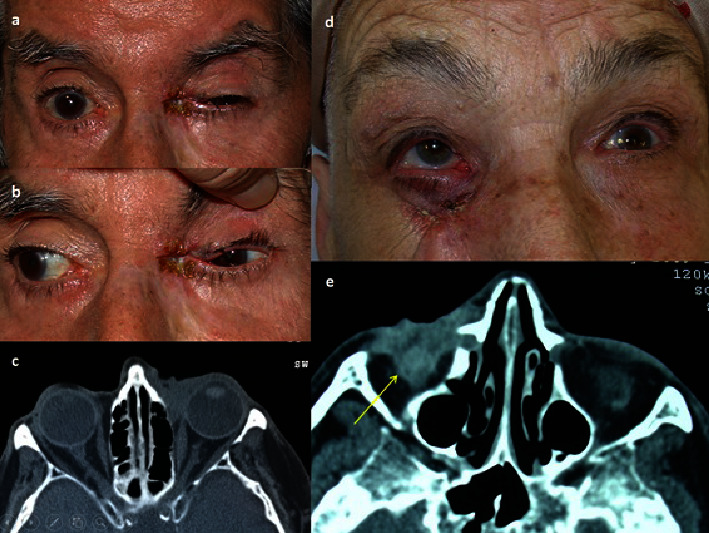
Recurrent basal cell carcinoma (BCC) (patient 11 in Table 1). (a) Ulcerative lesion in the medial canthus, adherent to deep planes. (b) Contraction of the eyelids that makes them difficult to open. Limitation in abduction of the eyeball. (c) Axial computed tomography scan demonstrates a soft tissue mass in the left inner of canthus of left orbit. The lesion shows homogeneous enhancement with slightly regular borders. The mass infiltrates the skin and subcutaneous layers, at the level of the inferior eyelid, involving the medial corner of the eye. There is infiltration of the medial inferior tarsal plate, of the anterior orbital tissue, and the anterior portion of the medial rectus muscle. There is no fatty plane of separation with the sclera (arrow). (d) Recurrent BCC (patient 7). Ulcerated mass in the lower periocular region, adherent to deep planes, and causing an upward displacement of the eyeball. (e) Radiographic confirmation of an irregular mass infiltrating the inferior orbital tissue, inferior rectus muscle, and orbital floor (arrow).
